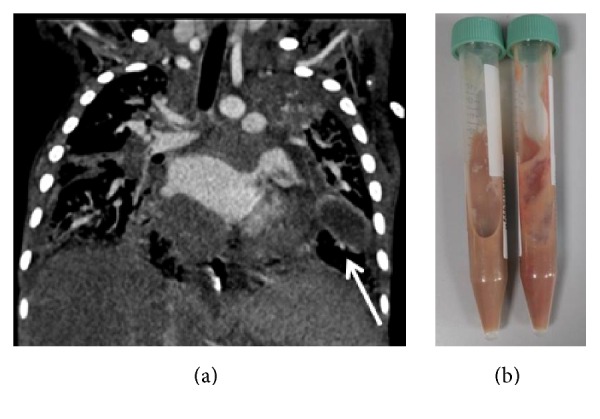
(a) Thorax CT coronal section: An abscess of 4.5 × 1.7 cm diameter in left inferior lobe in the neighborhood of cardiac apex (arrow). Parenchymal consolidations with punctate calcifications persevere. (b) Macroscopic appearance of the drainage material.
